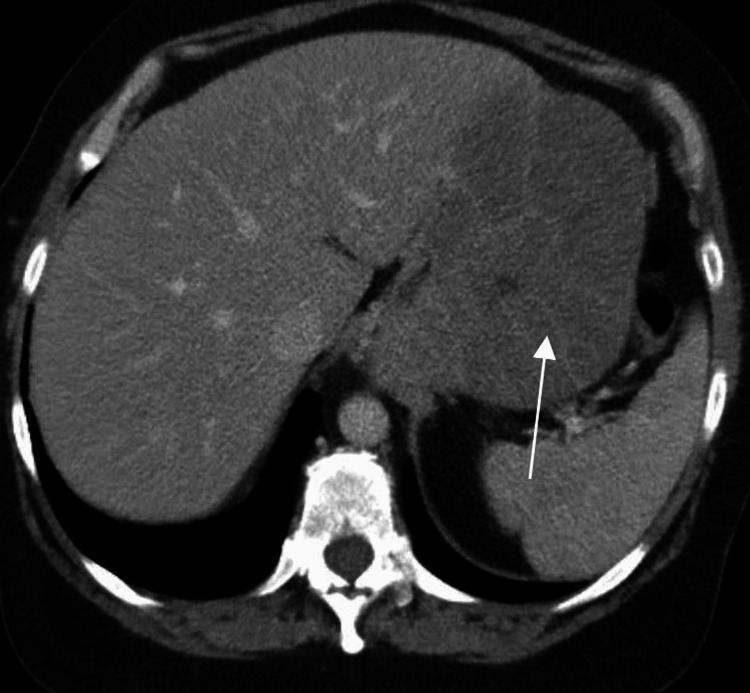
Axial IV contrast-enhanced CT image demonstrates marked rugal fold hypertrophy (white arrow)
